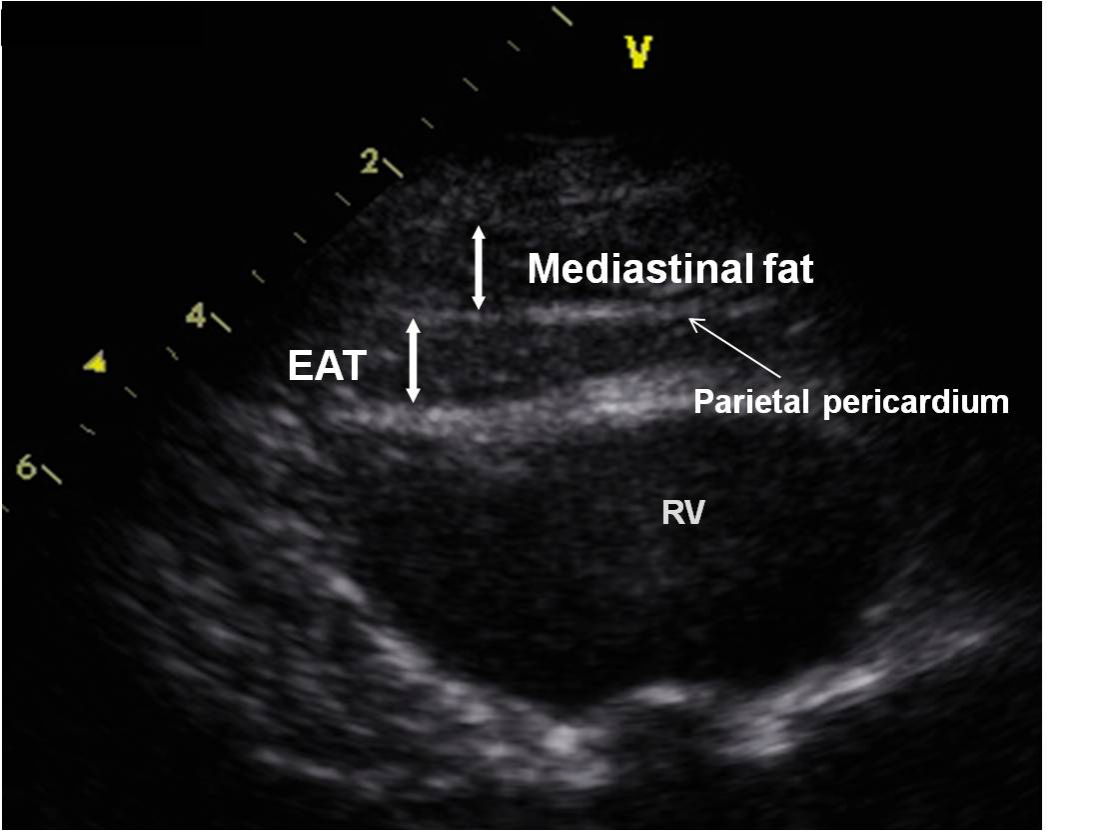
Echocardiographic measurement of epicardial adipose tissue thickness. Parasternal long-axis view at the mitral valve level. EAT, epicardial adipose tissue; RV, right ventricle
